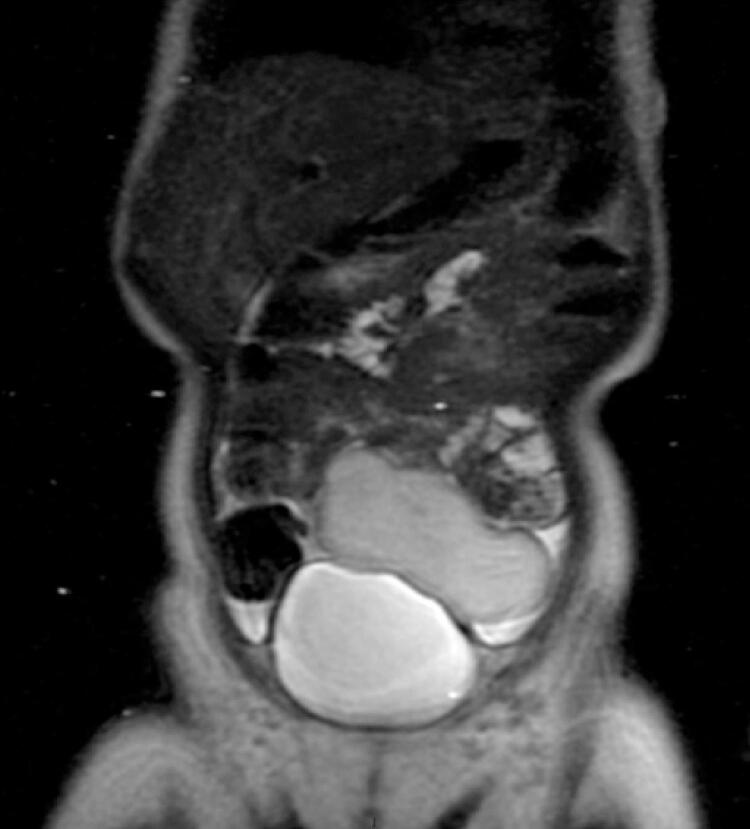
Coronal T2-weighted magnetic resonance imaging without gadolinium shows a mass with isosignal, discrete lobulated and well delimited, without infiltrative aspect, in close contact with the upper bladder wall and with the anterior surface of the peritoneum, displacing the upper intestinal loops and sigmoid colon, located in the meso/hypogastrium, extending to the left iliac fossa, and measuring 7.5x3.2x2.5cm
